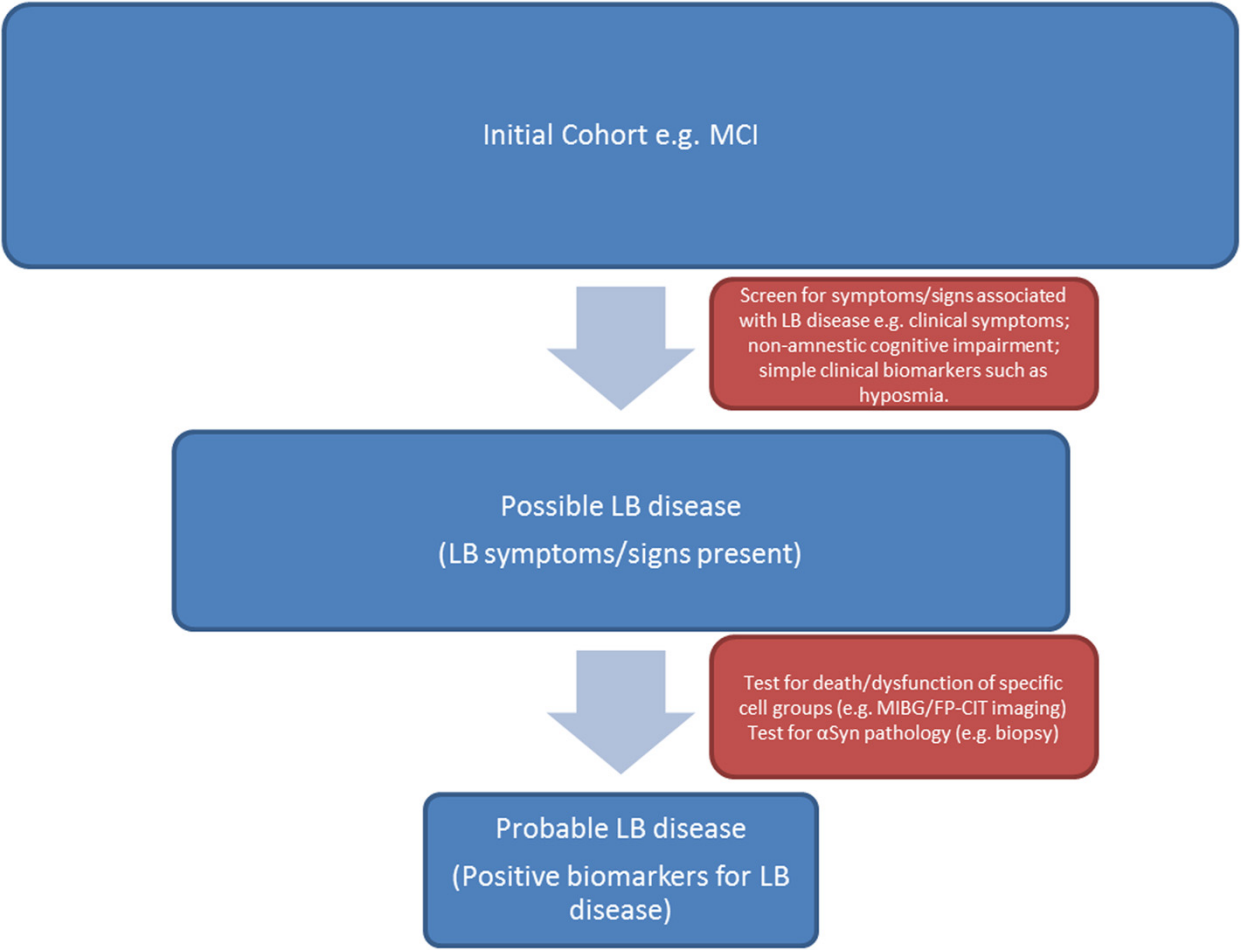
Hypothetical algorithm for the identification of prodromal Lewy body disease. The first stage consists of assessment for simple markers of functional decline – symptom questionnaires, cognitive tests and simple clinical biomarkers (for example, biomarkers for hyposmia). In the second stage, those with a profile suggestive of Lewy body (LB) disease would undergo tests with greater specificity, identifying death or dysfunction of cell groups affected by LB pathology, or the presence of alpha-synuclein (αSyn) pathology. This may include cardiac metaiodobenzylguanidine (MIBG) scintigraphy, measures of substantia nigra pathology, such as ultrasound or striatal dopamine transporter imaging, or biopsy for the presence of αSyn. FP-CIT, *N*-ω-fluoropropyl-2β-carbomethoxy-3β-(4-iodophenyl)nortropane; MCI, mild cognitive impairment.
